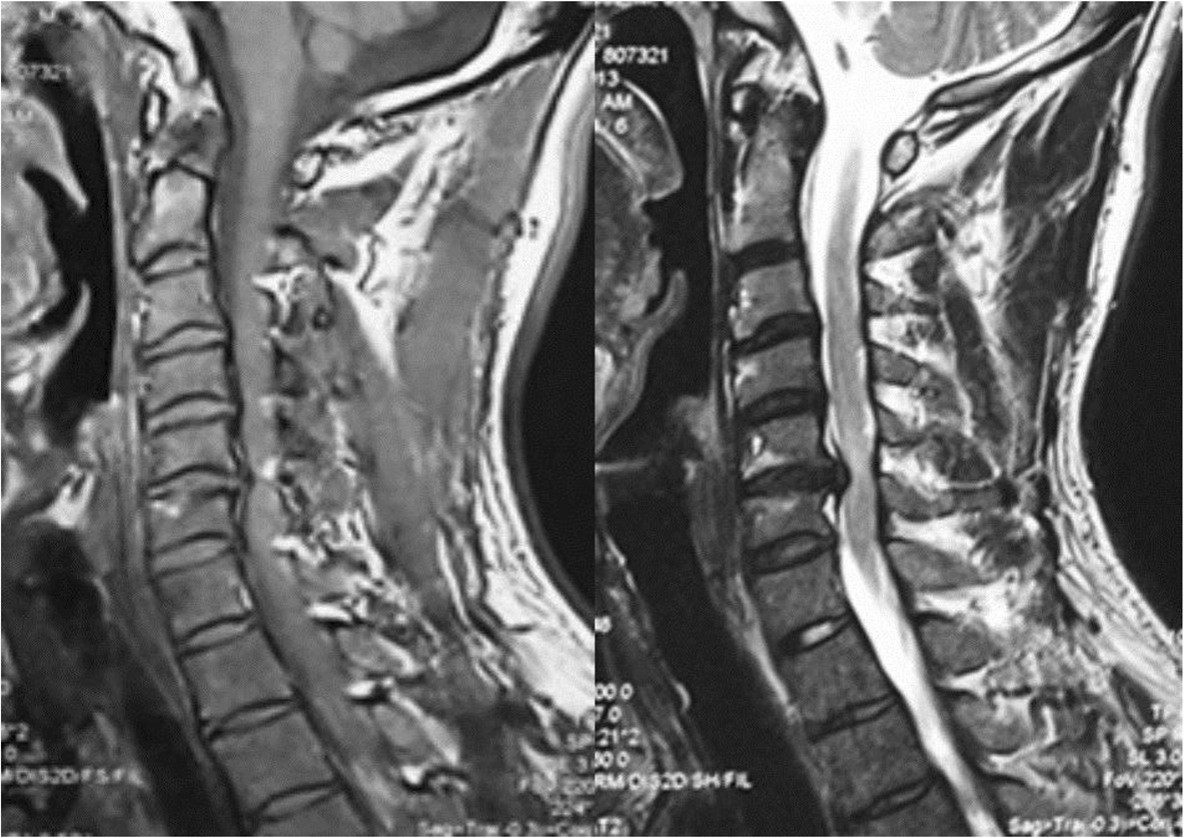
T1-weighted (left) and T2-weighted (right) images demonstrate C5-C6 with Modic-2 changes on MRI.
